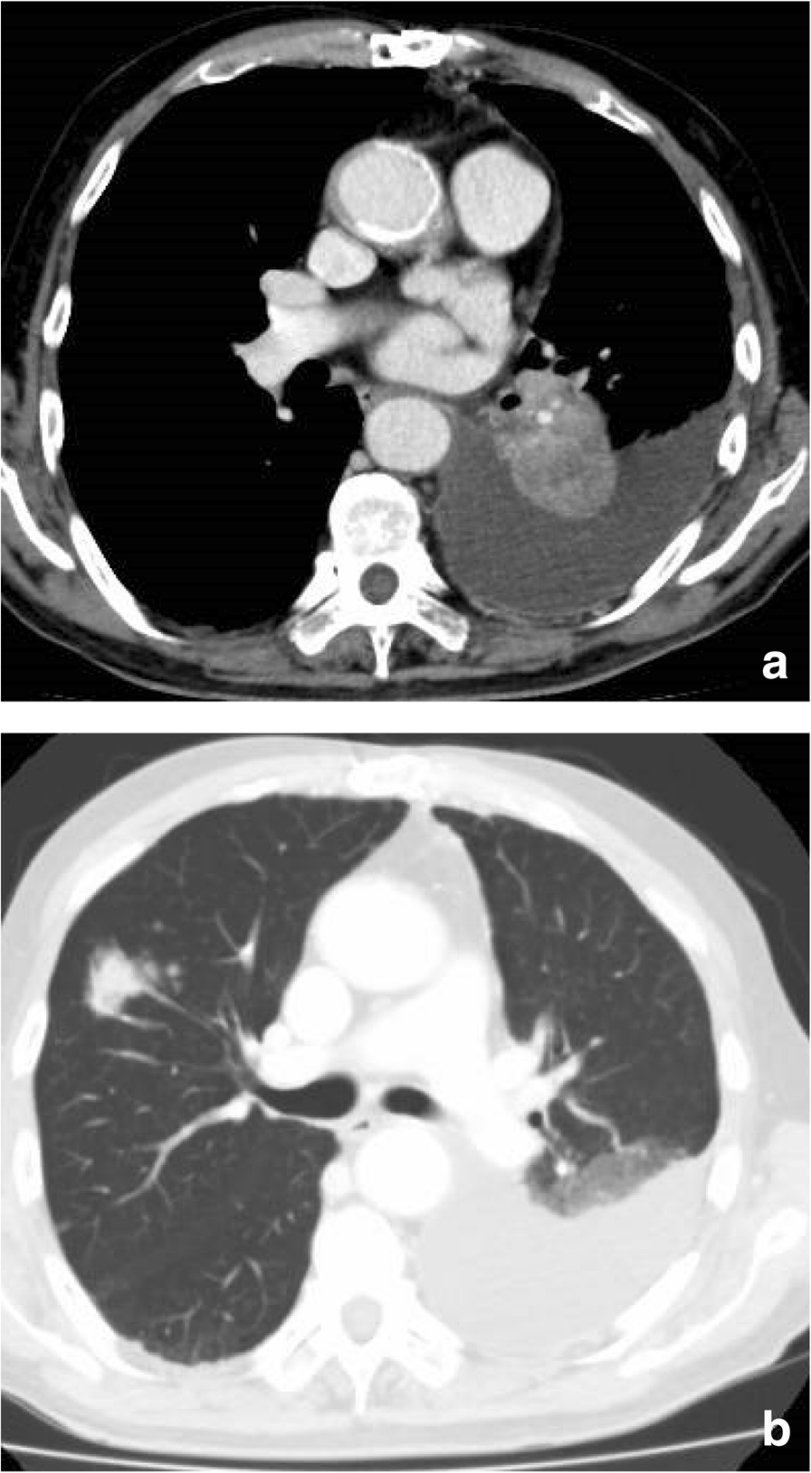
CT scan of the chest. CT scan of the chest, showing left hilar lung mass and left pleural effusion (a) and multiple lung nodules in the right lung (b)
